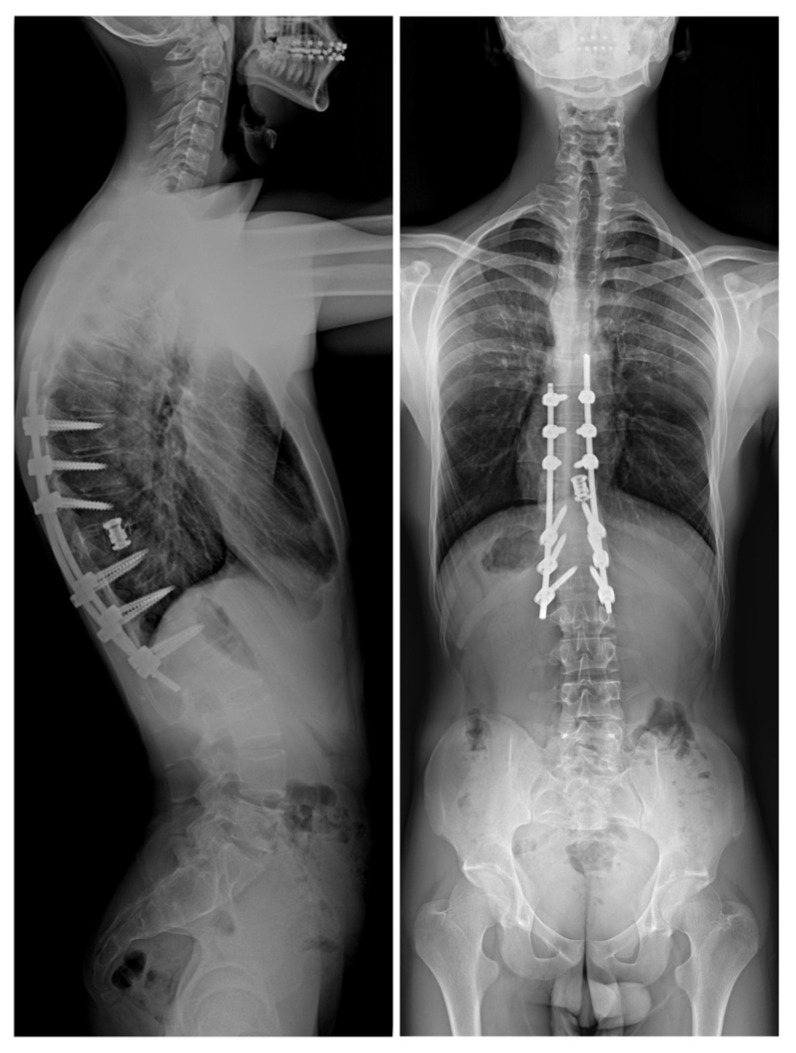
Anteroposterior (AP) and lateral (LAT) radiographs obtained 3 years after surgery, demonstrating maintained spinal correction, stable implant position, and satisfactory coronal and sagittal alignment. No evidence of hardware failure, loss of correction, or other postoperative complications was observed during follow-up.
